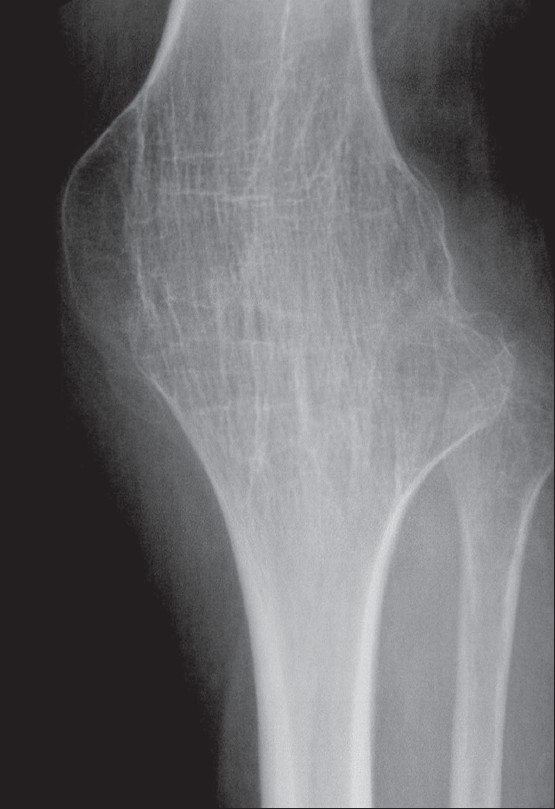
Plain radiograph shows bony ankylosis of the left knee, secondary to prior surgical intervention for end-stage destructive tuberculous arthropathy
